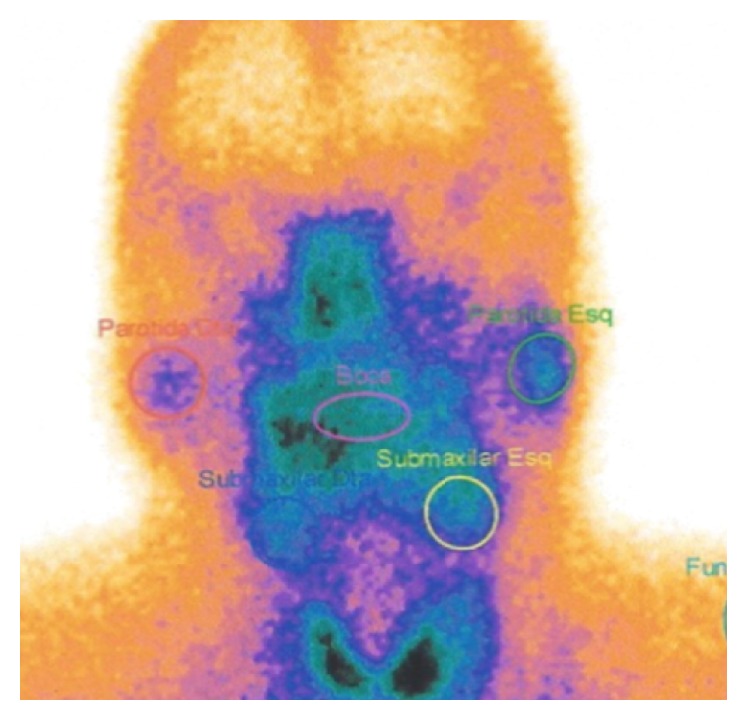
Salivary gland scintigraphy showing decreased uptake in submaxillary and parotid.
